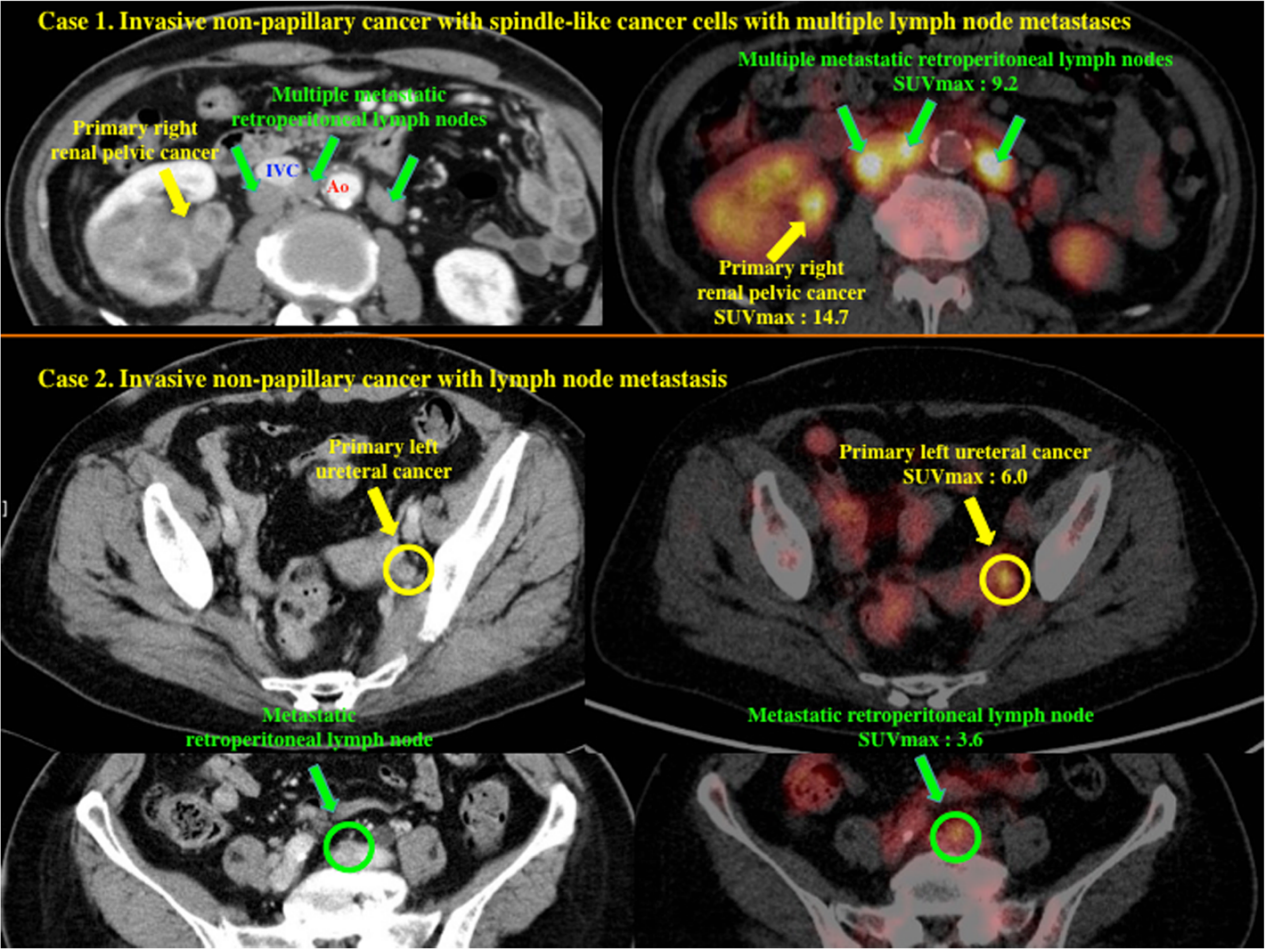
Representative images of PET-CT Case 1 presented with primary right renal pelvic tumors and multiple retroperitoneal lymph nodes involvement. Case 2 presented with primary left ureteral tumors with metastatic retroperitoneal lymph node. Ao; abdominal aorta, IVC; inferior vena cava
